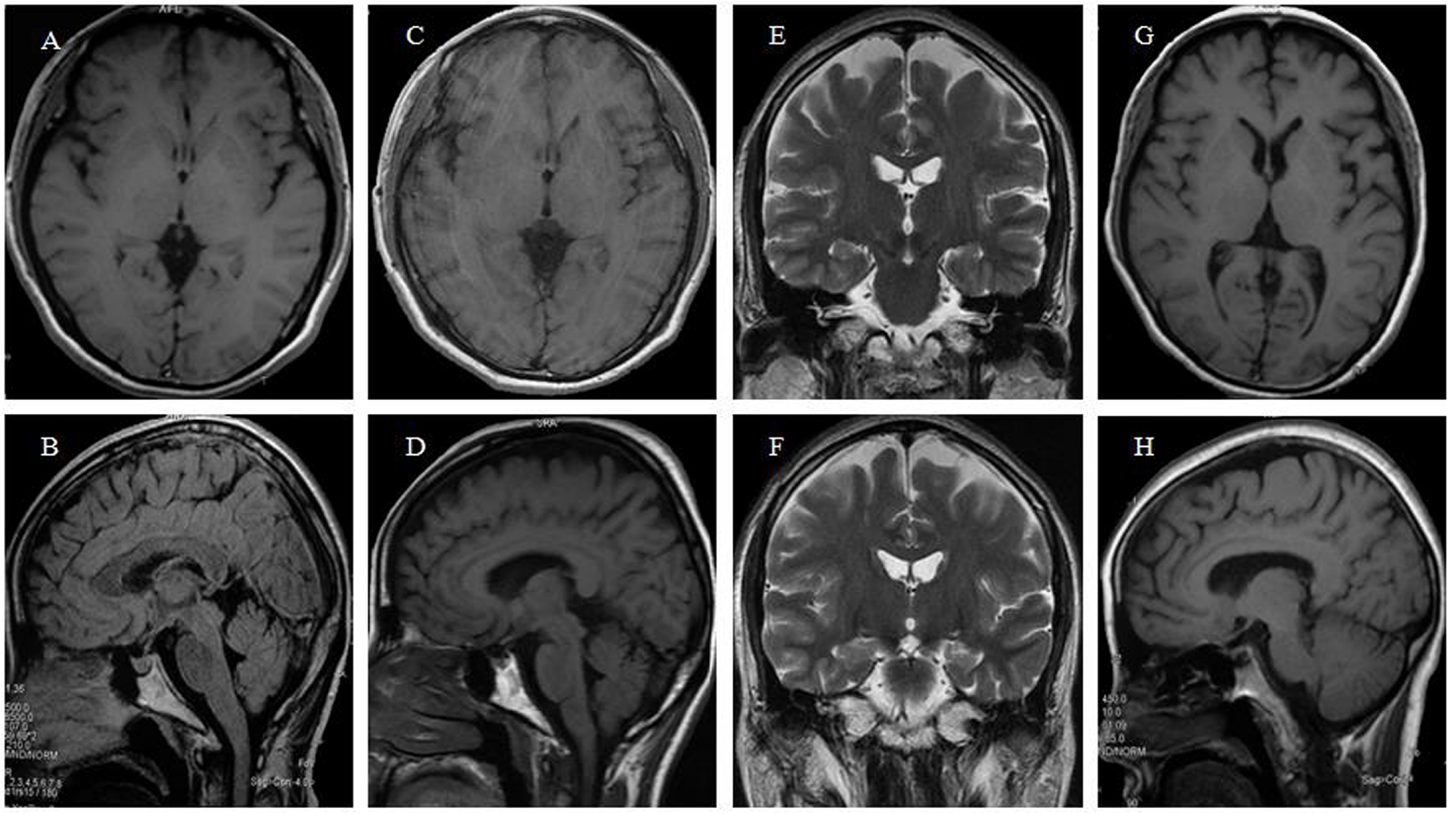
Brain MRI and CT scans of two patients of the family. A,B: brain MRI scan of the proband at the age of 42; C-F: brain MRI scan of the proband at the age of 44; G,H: brain MRI scan of the patient Ⅳ20 at the age of 46. Atrophy of whole brain was present in both patients. The frontotemporal atrophy was the most noticeable, while in the proband the hippocampus was relatively well-preserved.
